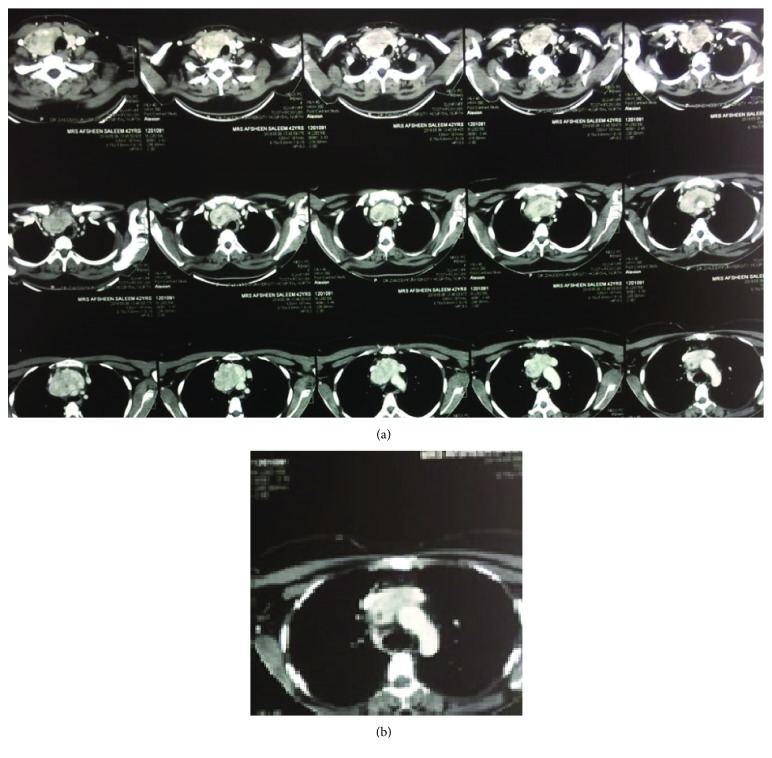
(a) Multiple axial sections of CT scan showing retrosternal extension of thyroid mass. (b) Axial section showing inferior end of mass lying over the arch of aorta.
